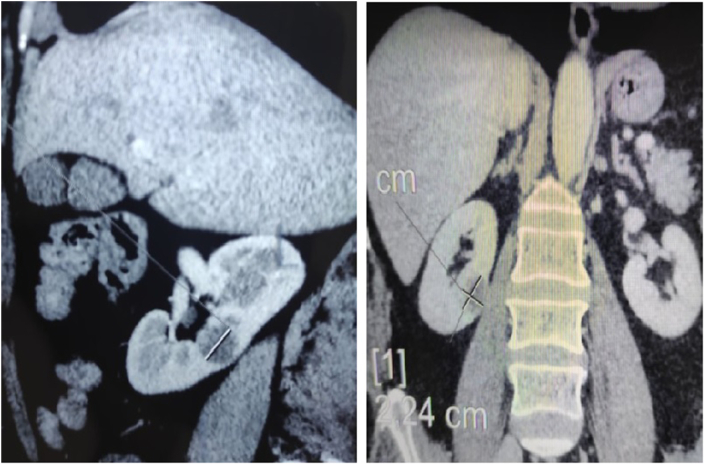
Plain abdominal computed tomography (CT) shows single right mediorenal solid-cystic mass measuring 22*24 mm, round and well limited.
